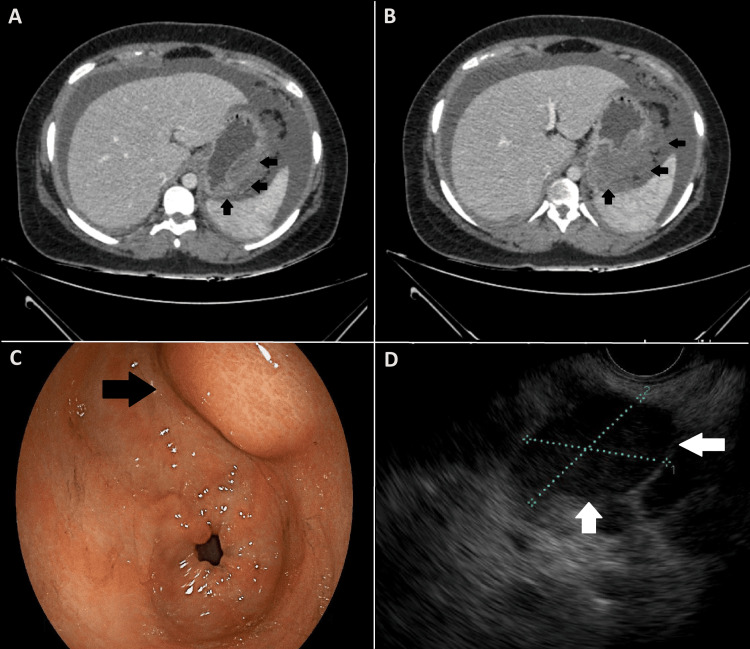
CT, EGDscopy, and EUS images of the NET A and B: A contrast-enhanced CT of the abdomen showing a large ill-defined heterogeneous hypodense mass lesion along the greater curvature of the stomach. C: EGDscopy showing a submucosal lesion along the greater curvature of the stomach. D: An EUS image showing a hypoechoic submucosal lesion. CT: computed tomography; EGDscopy: esophagogastroduodenoscopy; EUS: endoscopic ultrasound; NET: neuroendocrine tumor
